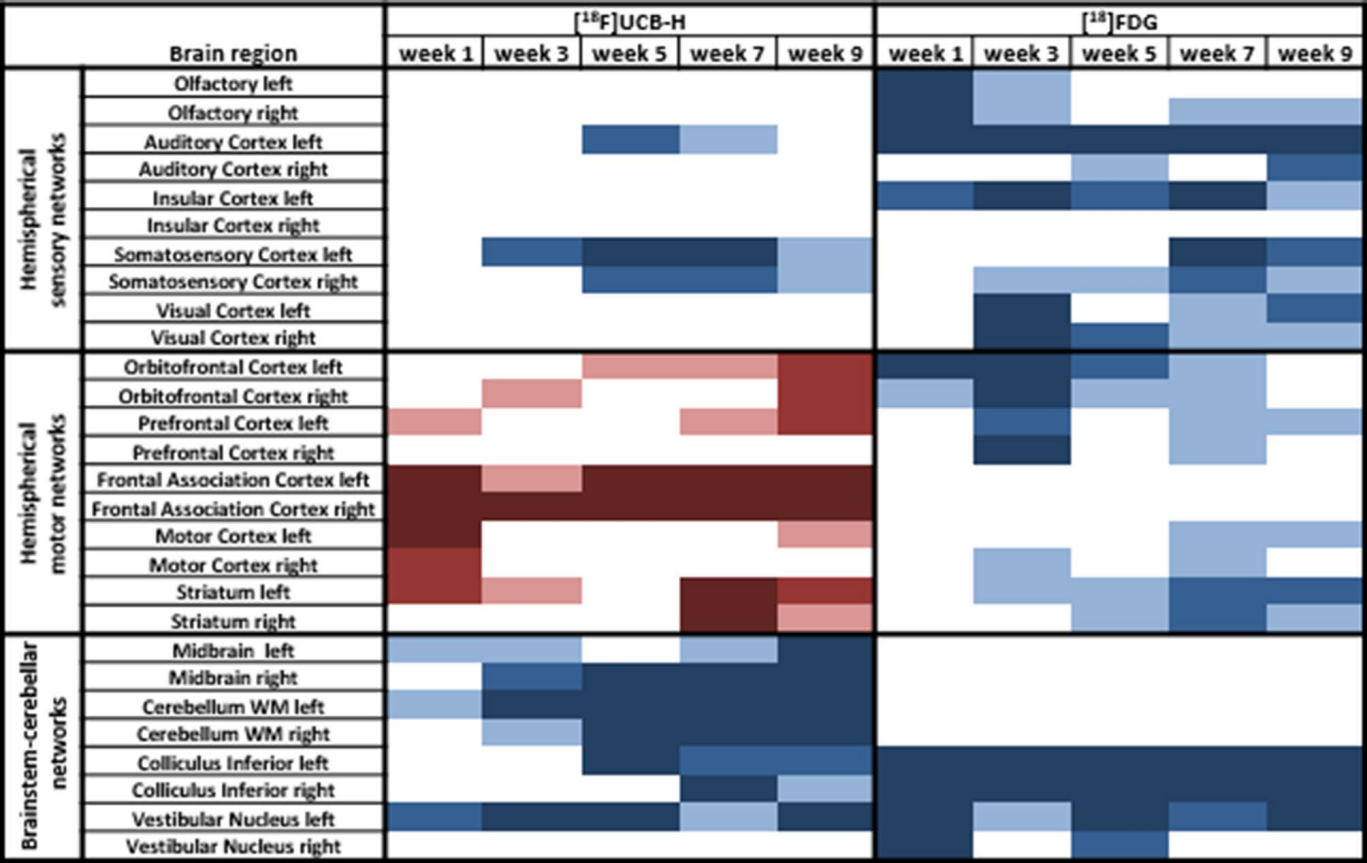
Overview of the changes in synaptic density measured by [^18^F]UCB-H PET (left side) and regional cerebral glucose metabolism (rCGM) measured by [^18^F]FDG PET (right side) in 28 selected brain regions, which are known to be involved in vestibular and sensorimotor processing. Mean normalized activity values per segmented brain region at 1, 3, 5, 7 and 9 weeks post bilateral labyrinthectomy (BL) were statistically compared using a one-way repeated measurement ANOVA with post-hoc paired t-tests and Bonferroni correction for multiple testing. Significant increases in synaptic density or rCGM are visualized in red scales (light red: *p* < 0.05, red: *p* < 0.005, dark red: *p* < 0.001), decreases in blue scales (light blue: *p* < 0.05, blue: *p* < 0.005, dark blue: *p* < 0.001). Comparison of [^18^F]UCB-H and [^18^F]FDG patterns shows a complex structure–function relationship across brain networks. [^18^F]FDG: [^18^F]fluorodeoxyglucose.
